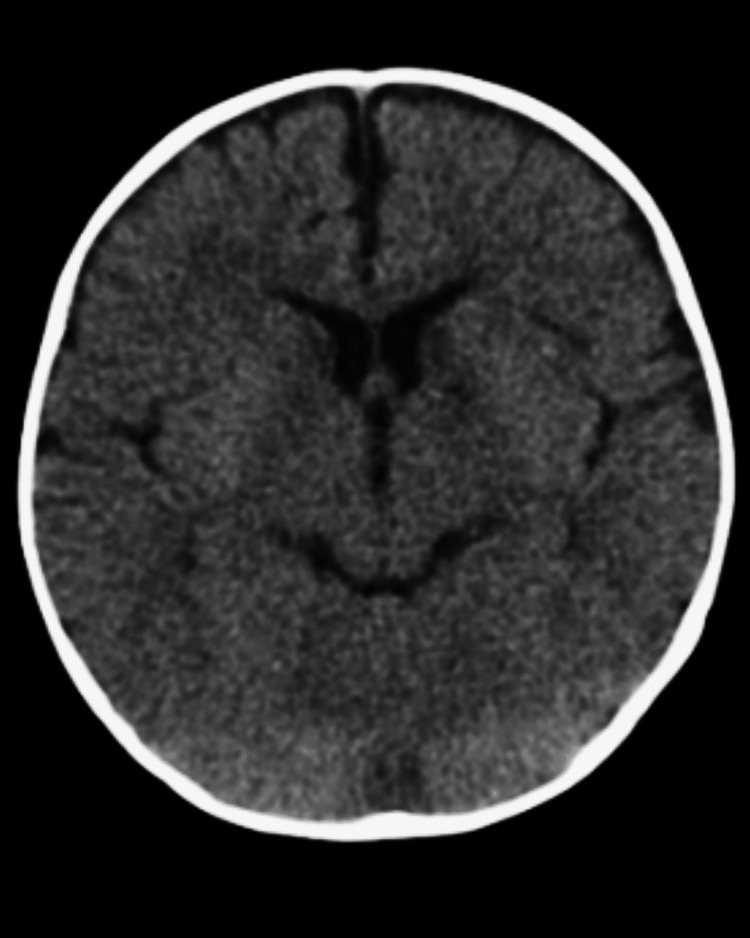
Initial axial non-contrast CT showing no acute intracranial abnormalities.
